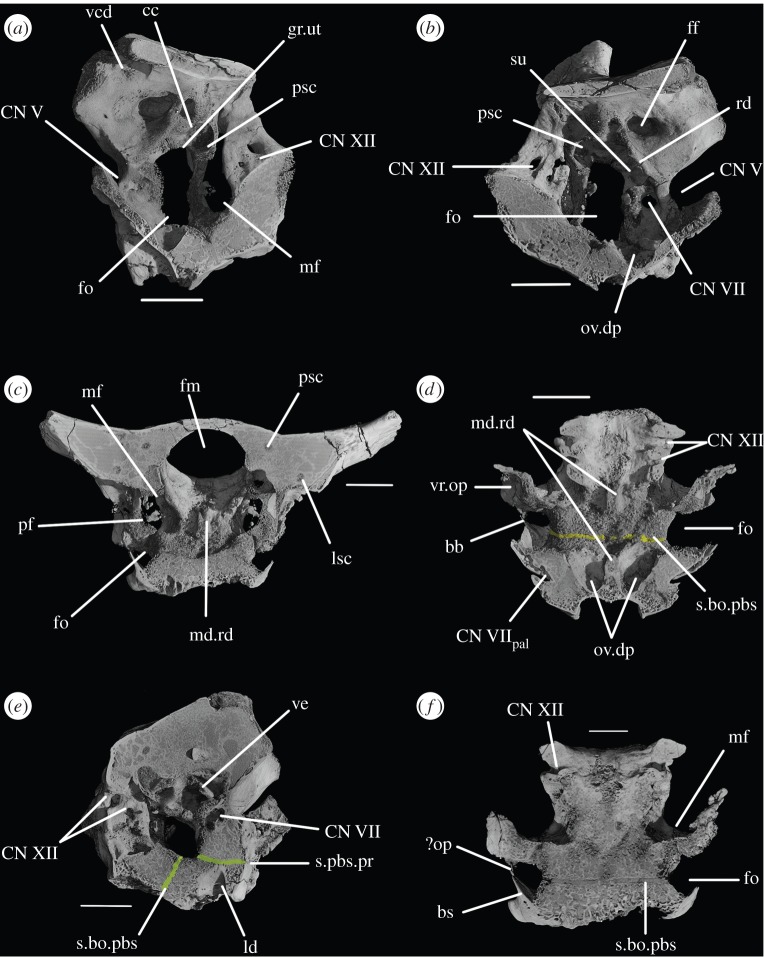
CT reconstructions of the braincase of SAM-PK-7696 (*a*) in right medial view, (*b*) in left medial view, (*c*) in anterior view (only posterior part showing, anterior cut off), (*d*) showing braincase floor in dorsal view, (*e*) in cross section to right of midline through opisthotic, to show basisphenoid contribution to ATR and (*f*) in cross section showing braincase floor in dorsal view, more ventral than (*d*), showing detail of basisphenoid posterior contact with ventral ramus of the opisthotic. For abbreviations, see table 1.
